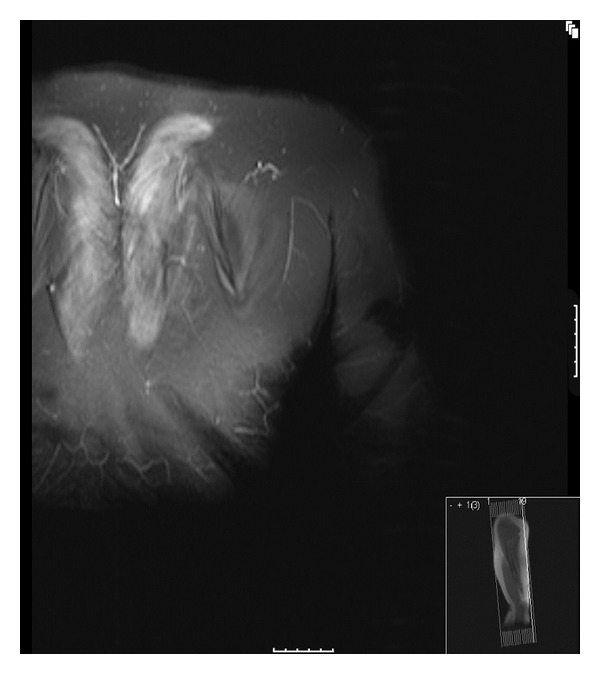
Bilateral increased intensity of the trapezius in the STIR sequence (MRI sagittal section in smaller box).
